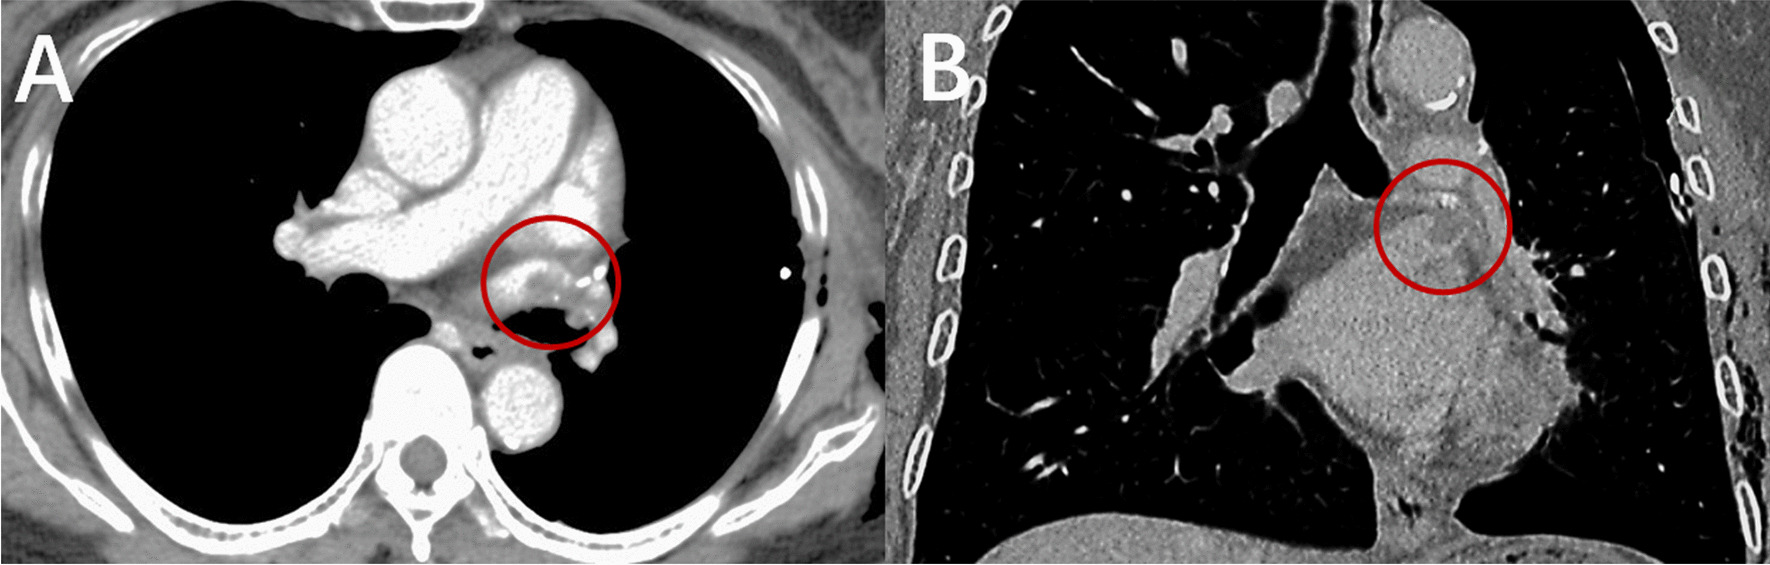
Typical radiological findings of thrombosis in the pulmonary vein stump after pulmonary lobectomy. a Thrombosis in the left superior pulmonary vein stump was detected by postoperative chest contrast-enhanced axial computed tomography scans; b Thrombosis in the left superior pulmonary vein stump was detected by postoperative chest contrast-enhanced coronal computed tomography scans
